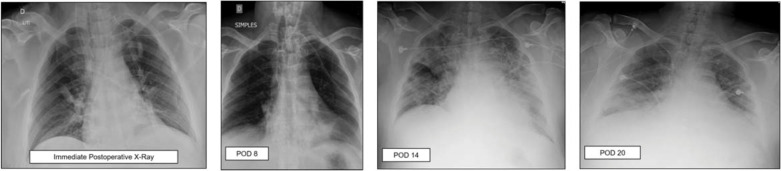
Evolution of chest X-rays over the days of hospitalization. POD=postoperative day.
